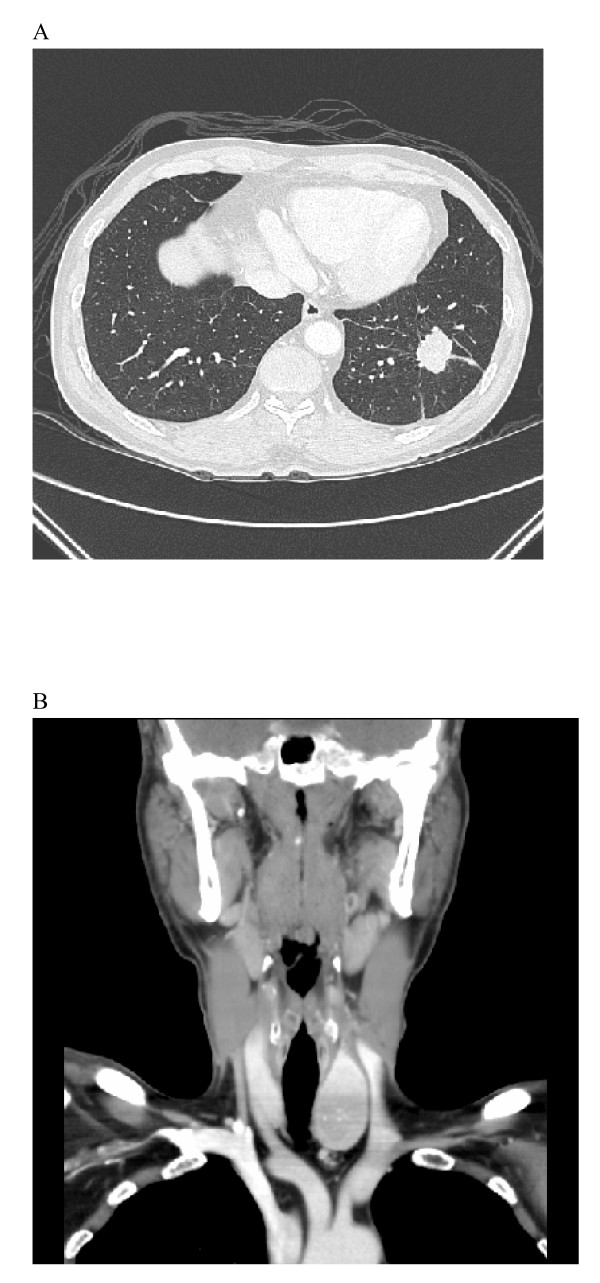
Computed tomography (CT) showing a nodule, 3.5 cm in diameter, within the left lower lobe of the lung with pleural retraction (A), and a mass 5 cm in diameter within the left lobe of the thyroid (B).
